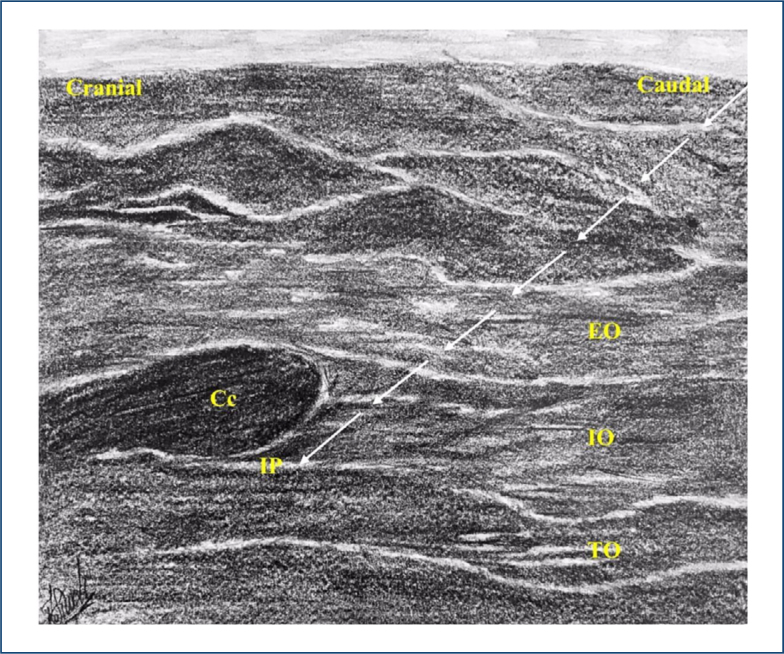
Modified thoracoabdominal nerves block through perichondrial approach block; reverse ultrasound anatomy. Cc: costal cartilage; EO: external oblique muscle; IO: internal oblique muscle; TO: transversus abdominis muscle; IP: injection point.
